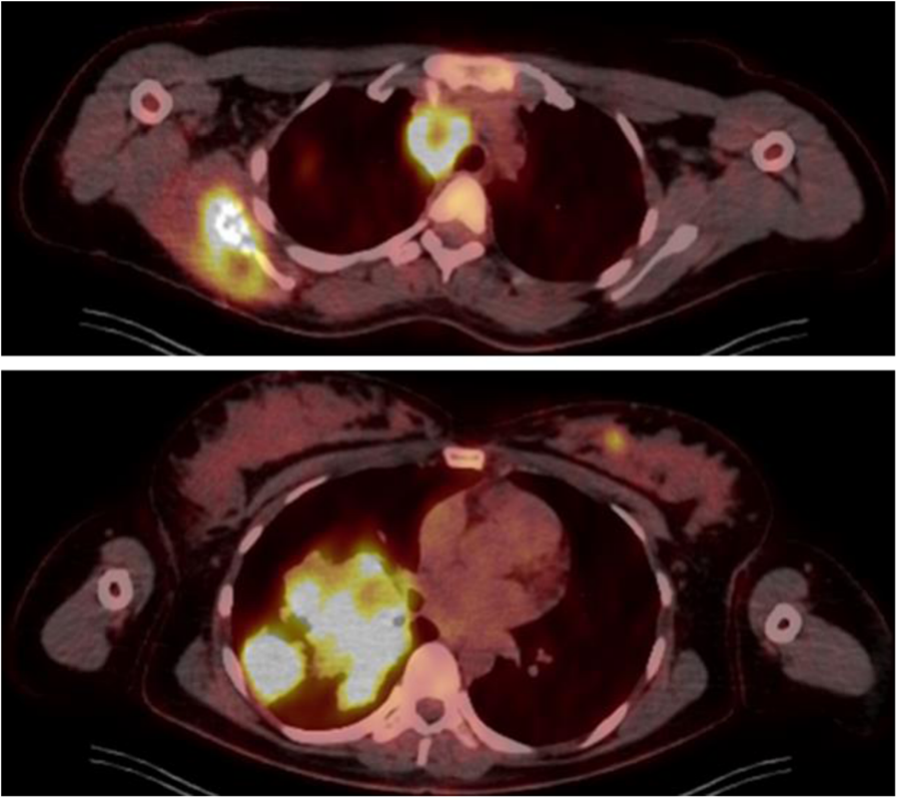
PET-CT axial view showing tumor lesions in the right scapula, mediastinum, and lung
